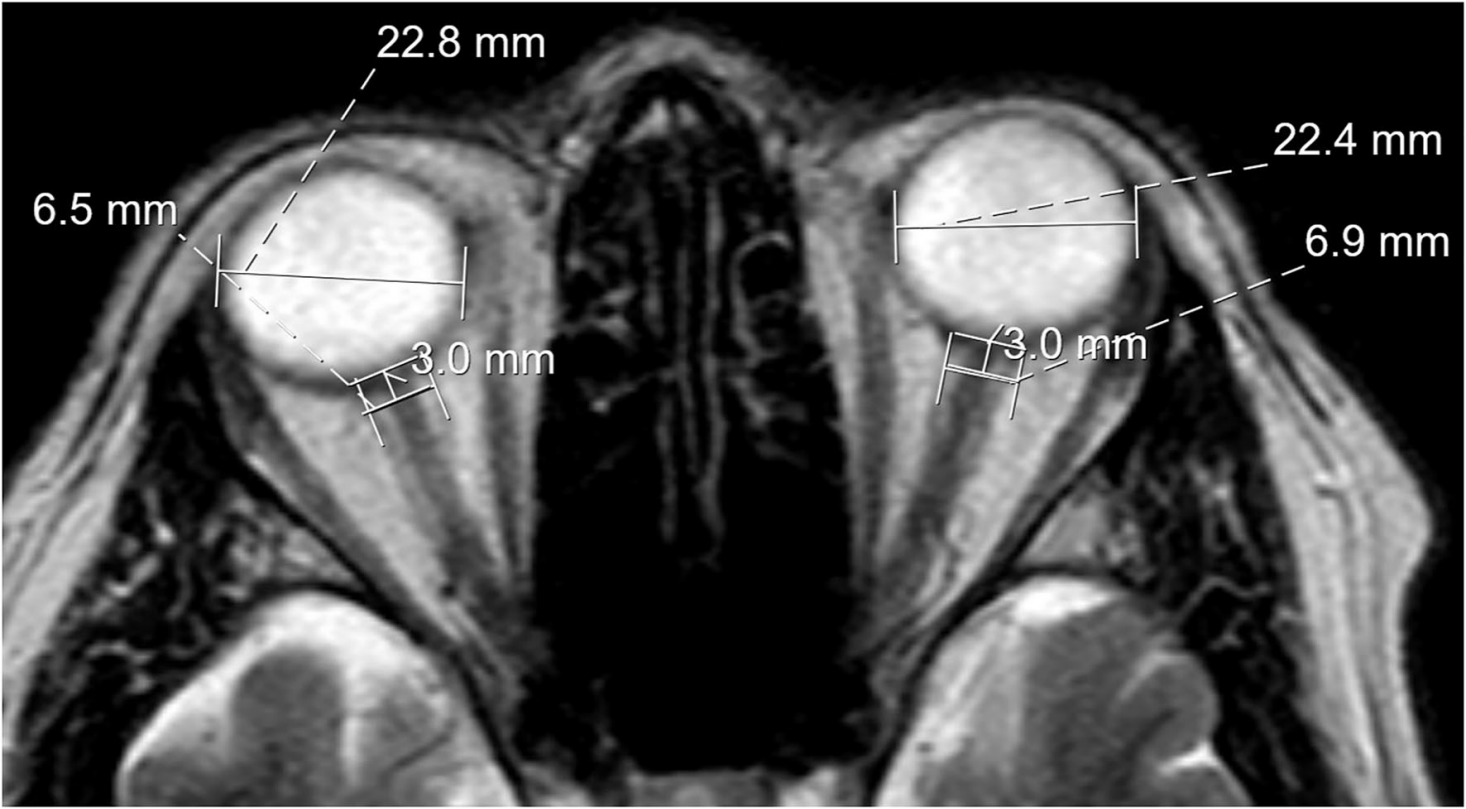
MRI scan demonstrating eyeball transverse diameter and optic nerve sheath diameter measurements, which, in this case, indicates abnormal widening.
